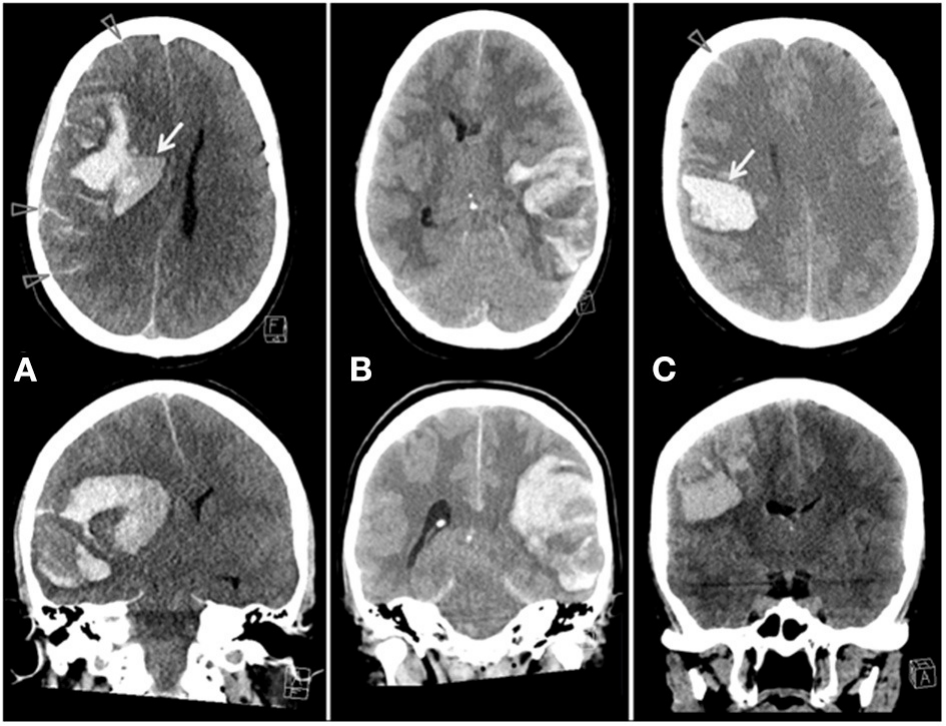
Axial and coronal CT from patient 1 (A), 2 (B) and 5 (C). These patients presented with large parenchymal hemorrhages. Note the fluid-fluid levels [arrows, (A,C)], the subarachnoid hemorrhage [arrowheads, (A,C)] and the heterogeneous appearance of all hematomas.
